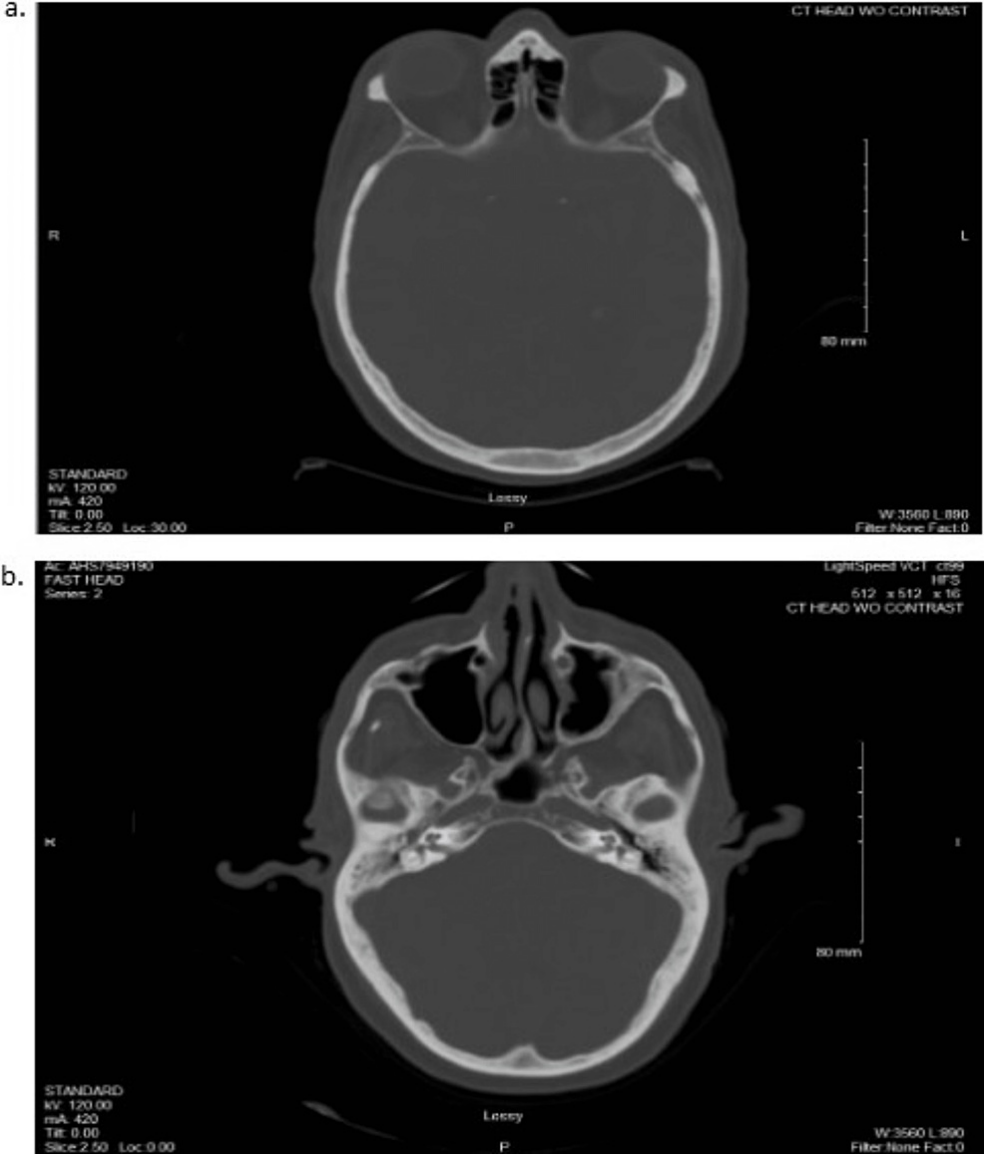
CT of the Head Without Contrast a: transverse plane, caudal; b: transverse plane, rostral CT: computed tomography
